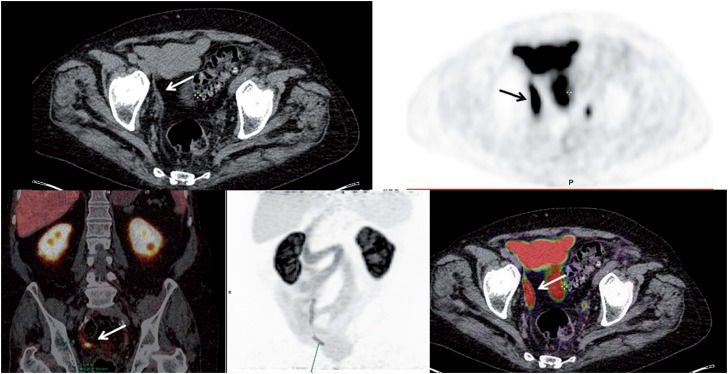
Anomalous accumulation of PSMA-68Ga in the right vas deferens. That has increased dimension compared to the contralateral, suggestive of secondary neoplastic lesion found, considering the underlying disease.
